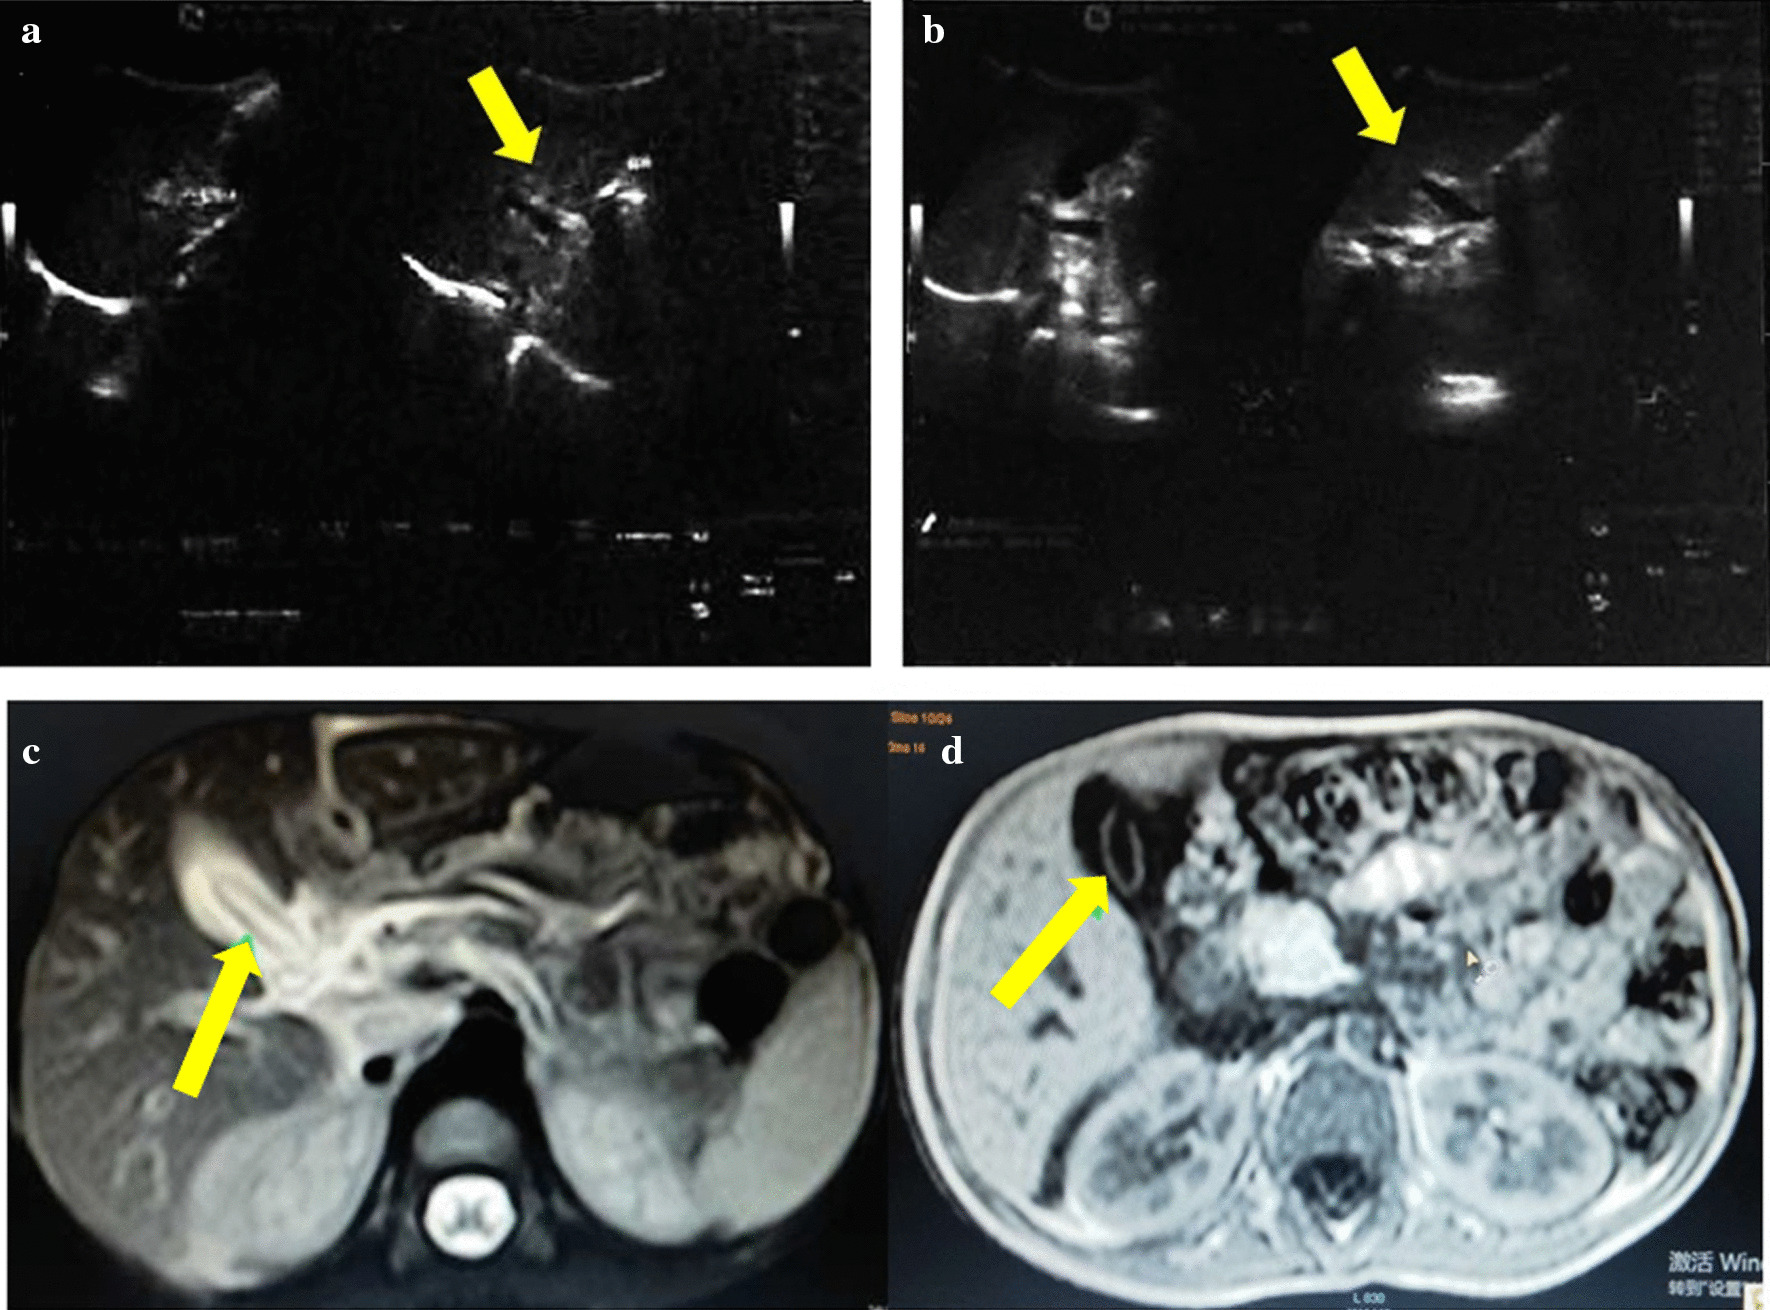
Ultrasonography and Magnetic resonance cholangiopancreatography image of Gallbladder ascariasis. a USG image showed structures (arrows) with hypoechogenic central and hyperechogenic edges with no acoustic shadow in the gallbladder suggested ascariasis. b There was no abnormal gallbladder size, and no abnormal echo in it, suggested no ascariasis in it. c, d T2-weighted axial magnetic resonance image showed a linear, hypointense band in the gallbladder (arrows), suggested AG.
